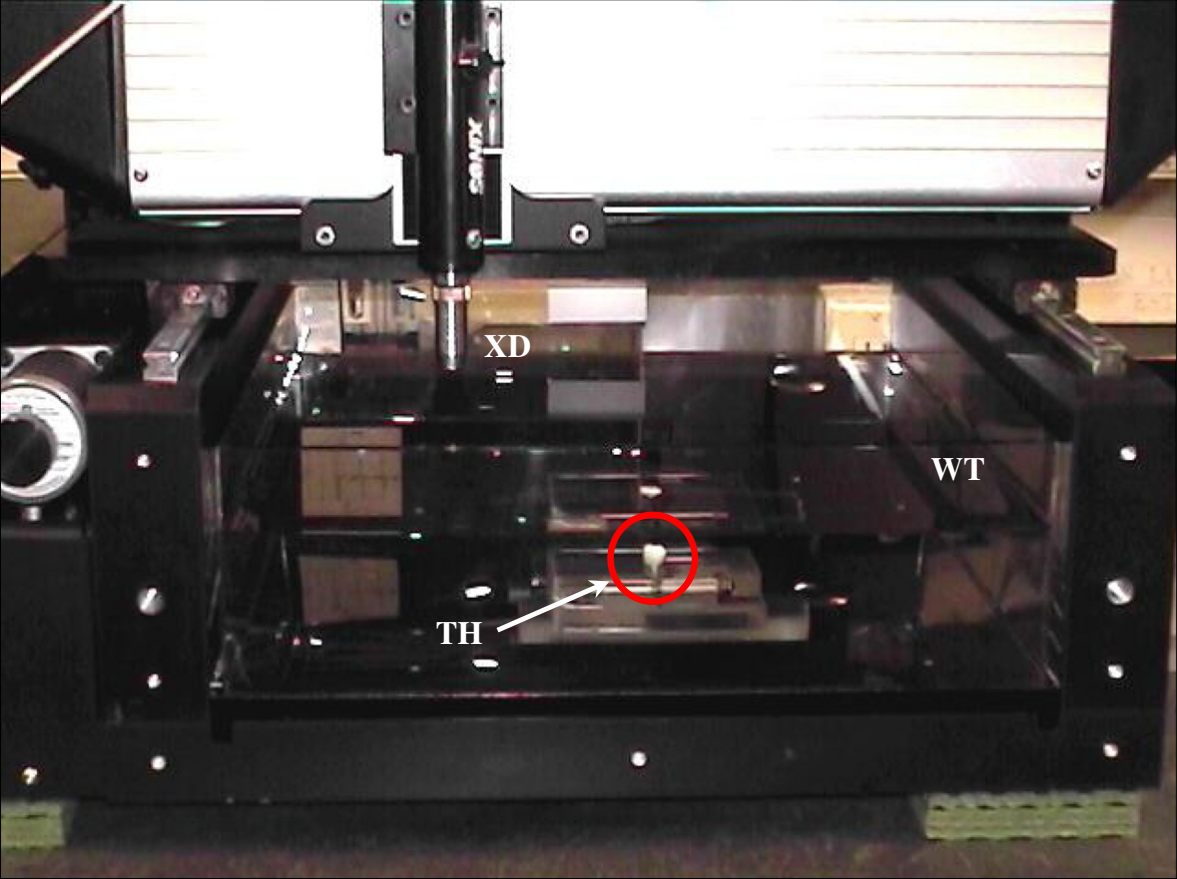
Experimental setup in the water tank. It shows the relative position of the ultrasound transducer (*XD*) and the tooth sample (*TH*; shown in *red circle*) in the water tank (*WT*).
